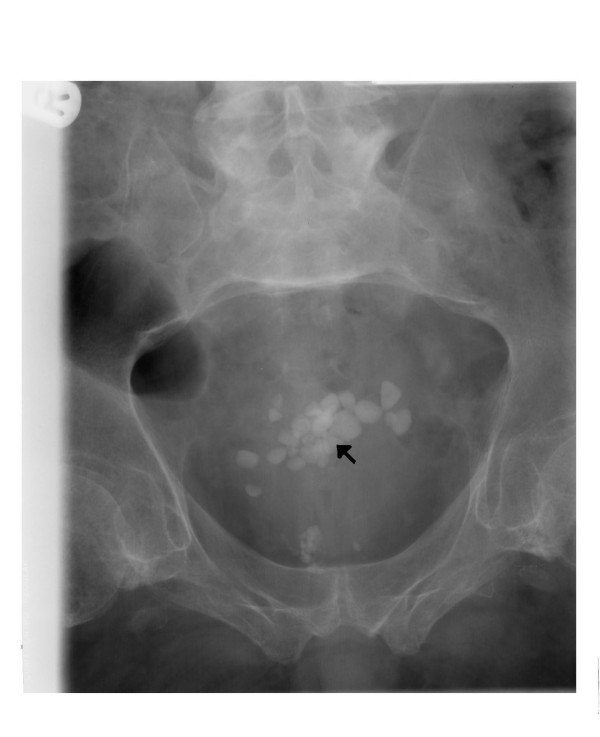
X-ray of urinary bladder taken on 14 May 2007 shows several vesical calculi (arrow).
